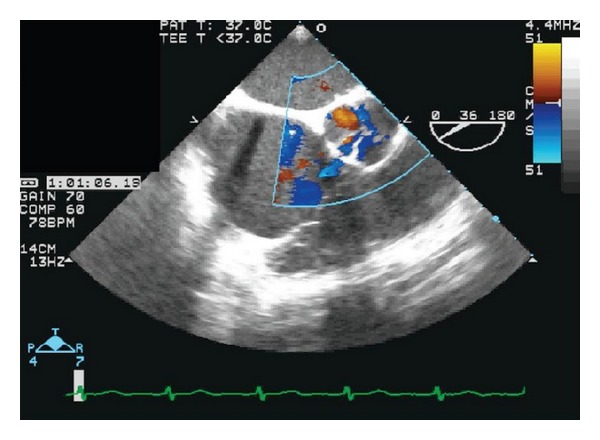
Transesophageal echocardiogram. Short-axis view shows more clearly the perforation and regurgitant jets. See Supplementary CINE file in Supplementary Material available online at http://dx.doi.org/10.1155/2013/528439.
